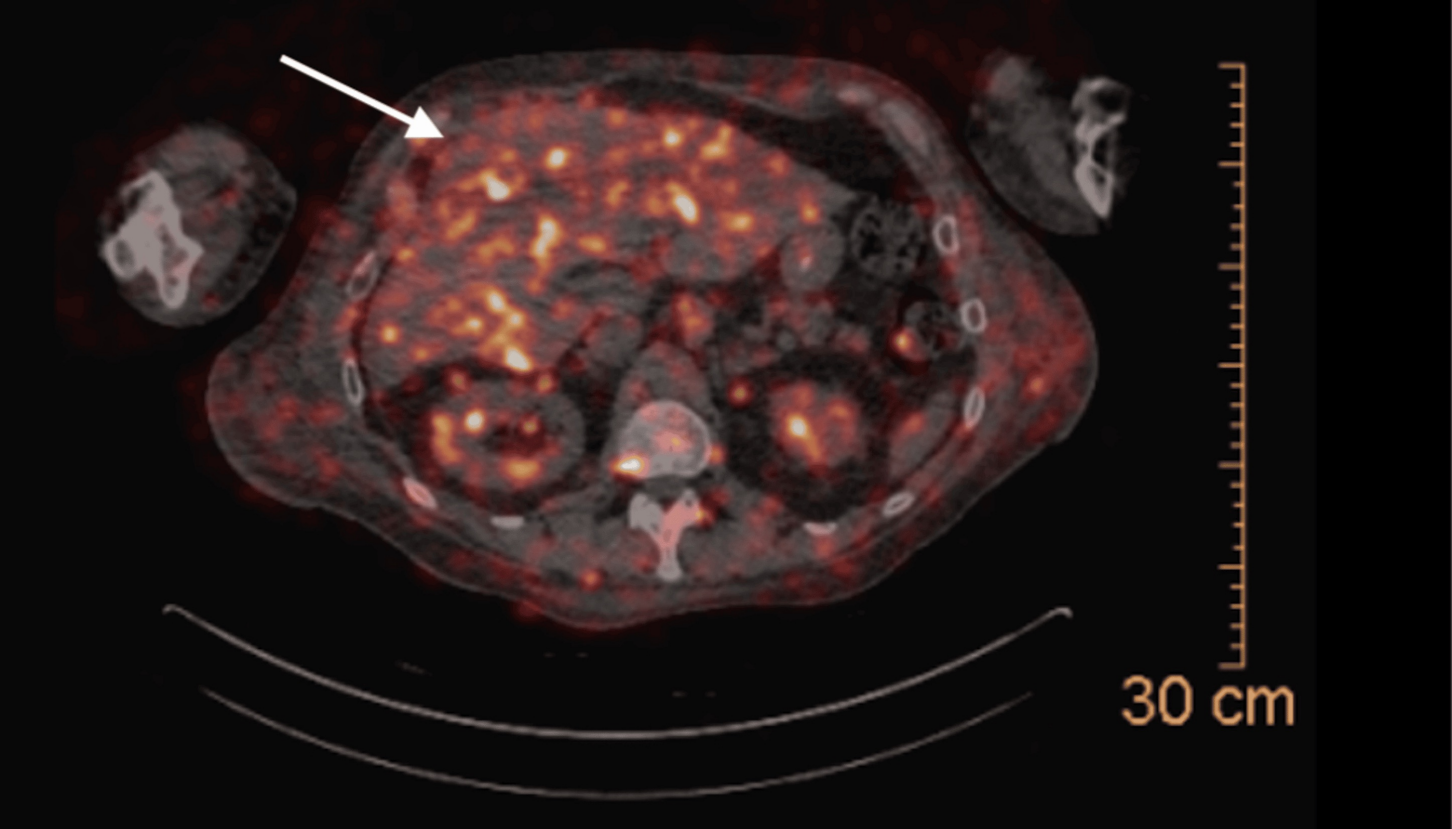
PET-CT showing FDG tracing in the heterogeneous liver. Arrow points toward the fluorodeoxyglucose (FDG) signals seen in the liver.
